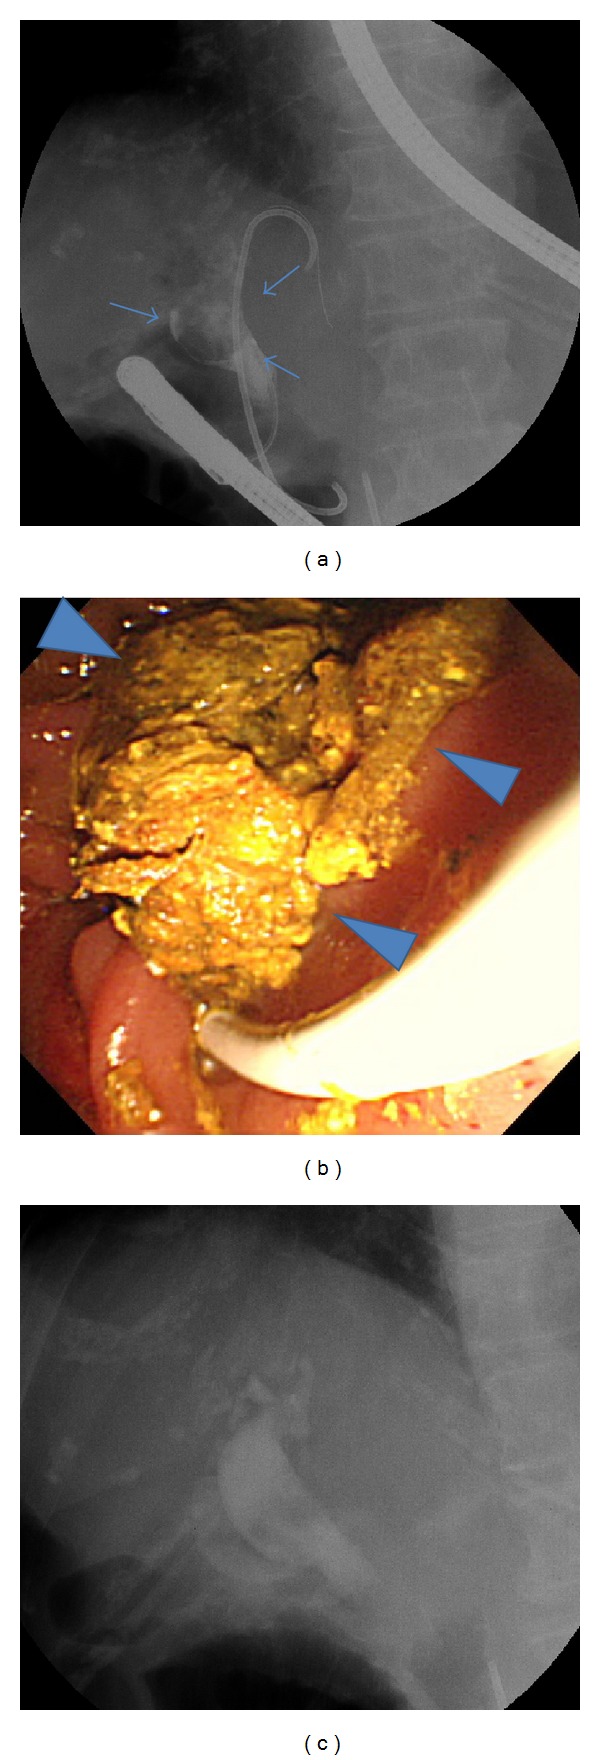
Endoscopic retrograde cholangiopancreatography (ERCP) images on hospital day 57. (a) Long-standing huge stone in the common bile duct (arrows). (b) A large amount of biliary sludge (arrowheads) and the stone, which had been difficult to remove, were washed out by a balloon catheter inserted to drain biliary sludge and infected bile (unable to photograph the stone). (c) Enhanced CT image showing no stone or biliary sludge remaining in the common bile duct after cleaning.
